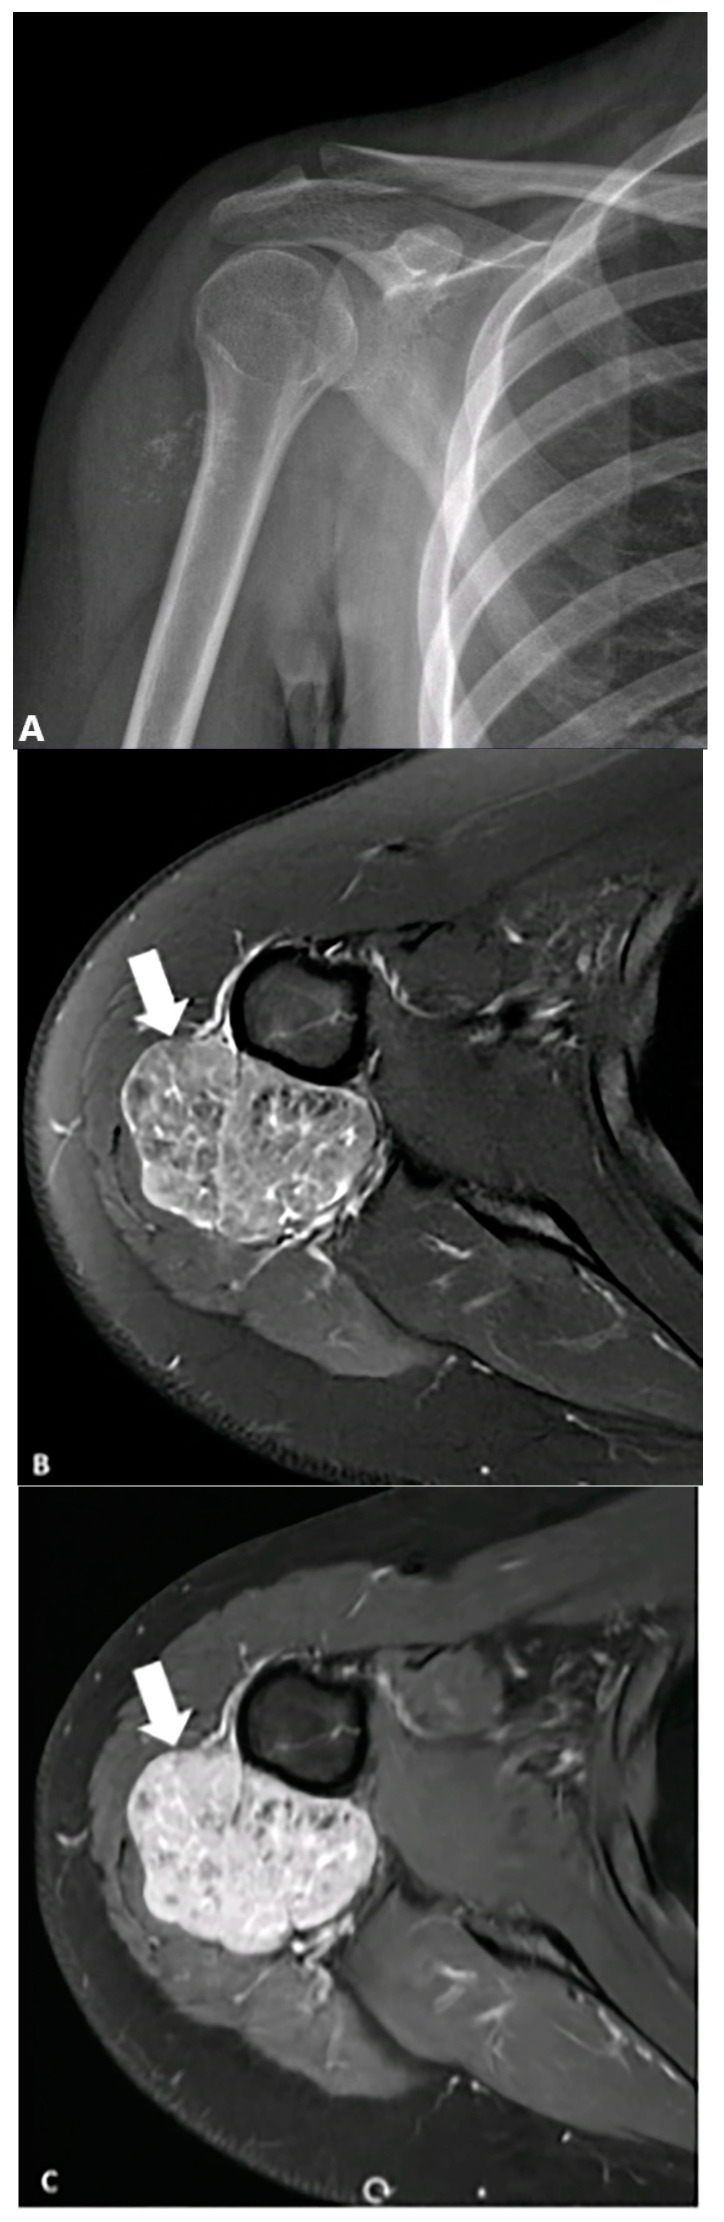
A 19-year-old female presented with a mildly tender, non-mobile mass in her right upper arm. (A) AP radiograph shows punctate calcifications concerning malignancy. (B) Axial fat-saturated T2-weighted MRI image demonstrates a heterogeneous lobulated mass (arrows) with hyperintense signal and hypointense foci corresponding to stippled (punctate) calcifications seen on the radiograph, which shows marked enhancement following contrast injection. (C) Biopsy confirms leiomyosarcoma. The image was obtained from the University of Washington Medical Imaging database with the required permissions.
